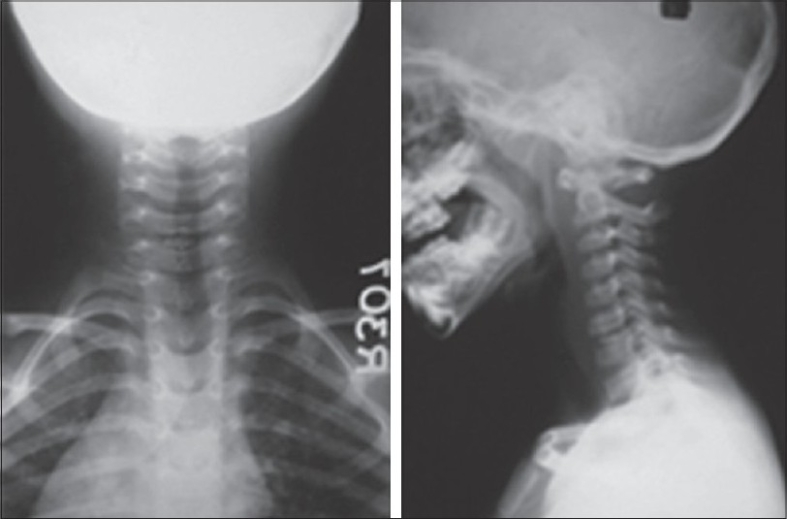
Cervical spine radiographs shows calcified disc at C6-C7 level
